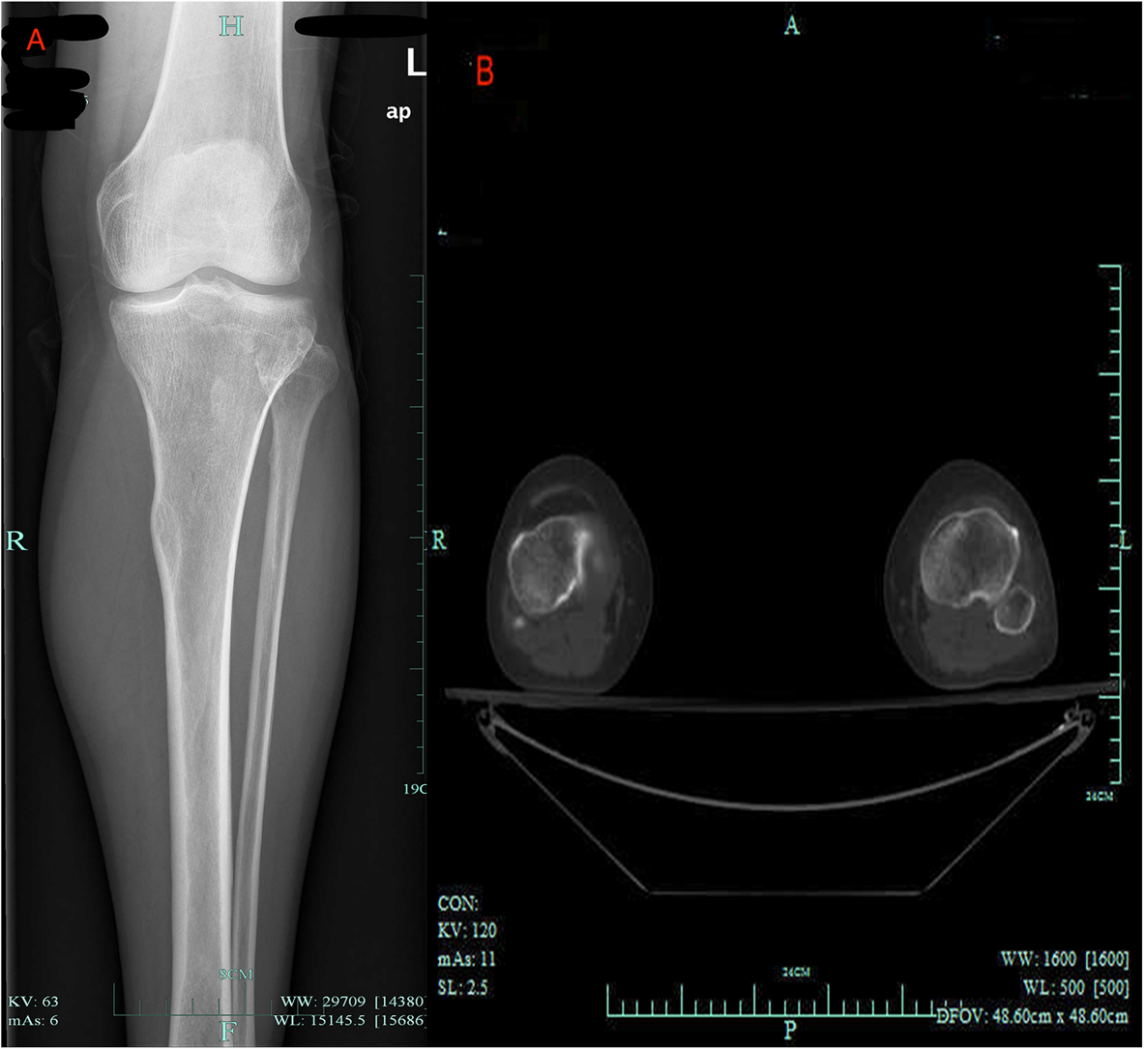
A X-ray documented no fracture. B Computed tomography (CT) documented no fracture
